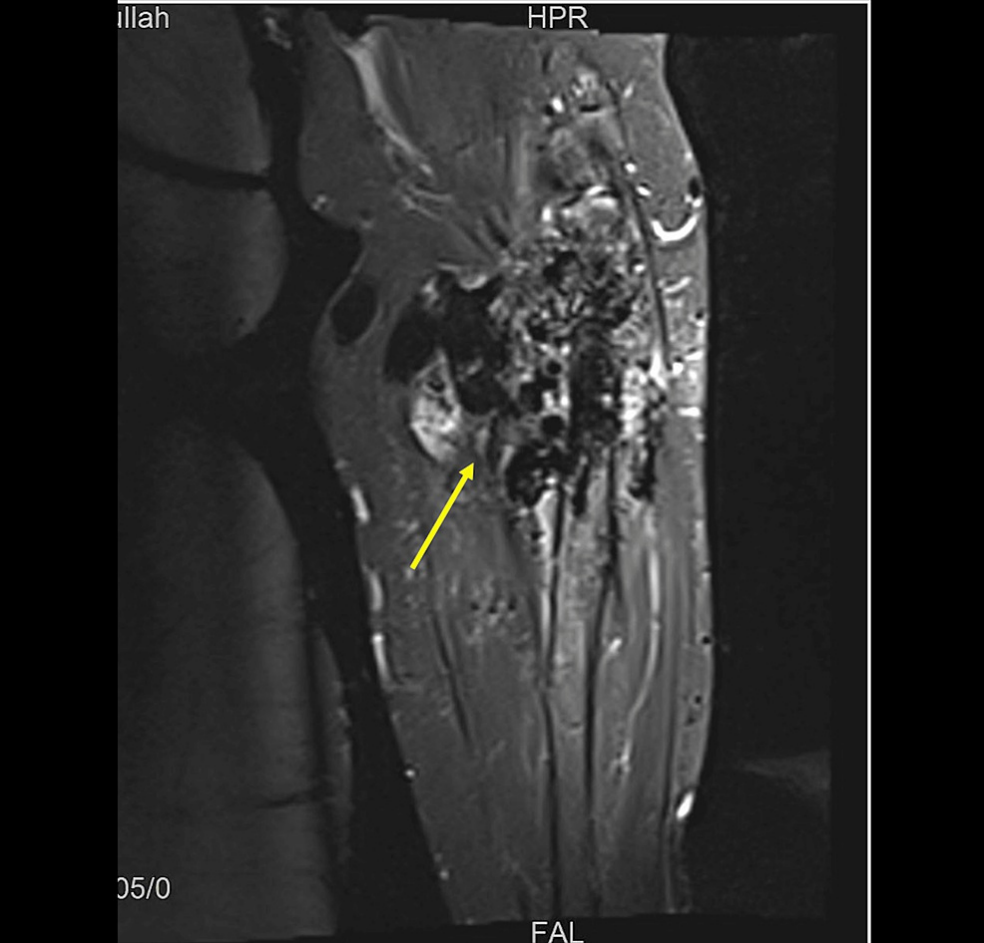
Magnetic resonance imaging (MRI) showing prominent forearm vessels
